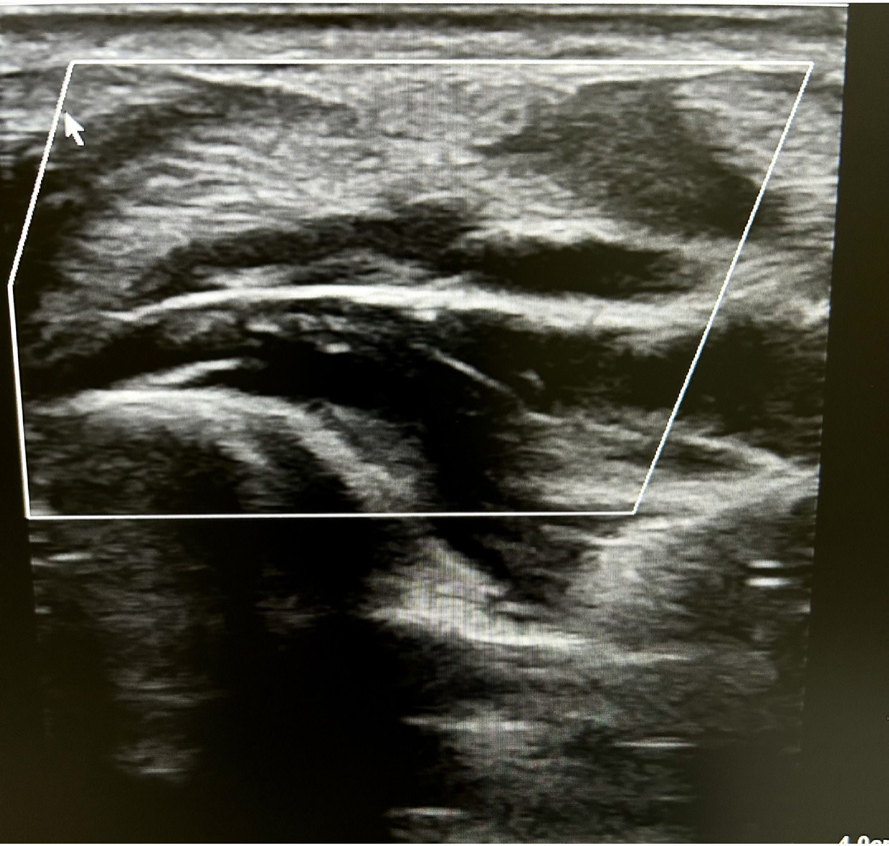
Absence of flow on Doppler along the path of the superficial ulnar artery.
